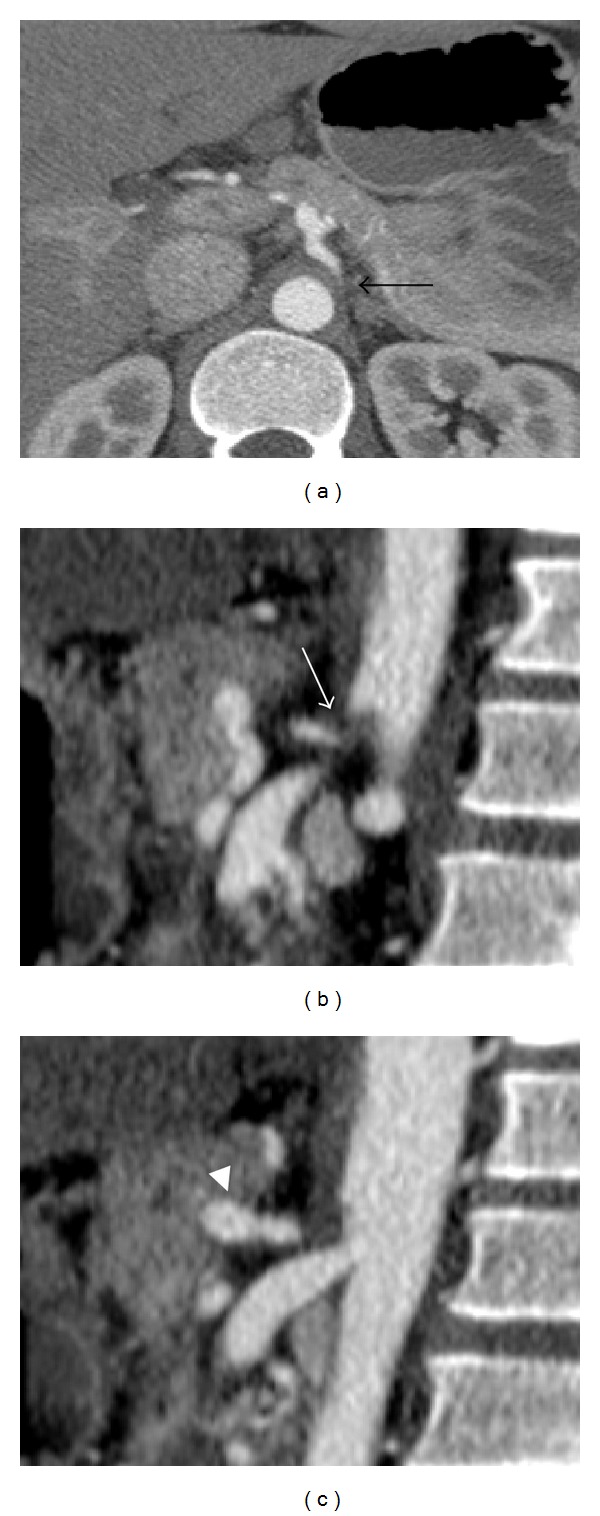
Axial (a) and sagittal (b and c) CT scans of MALS reveal severe stenosis of celiac axis from extrinsic compression (arrows) and poststenotic dilation of proximal celiac artery (arrowhead).
